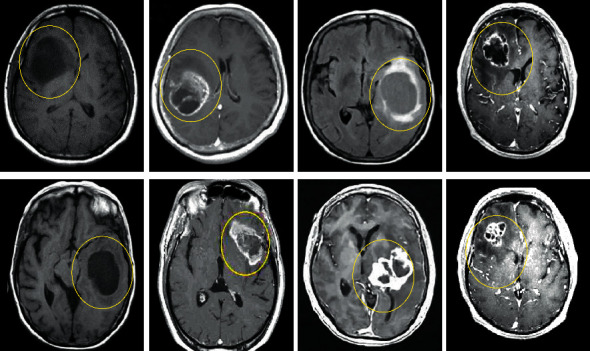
Magnetic resonance imaging results of glioma patients.
